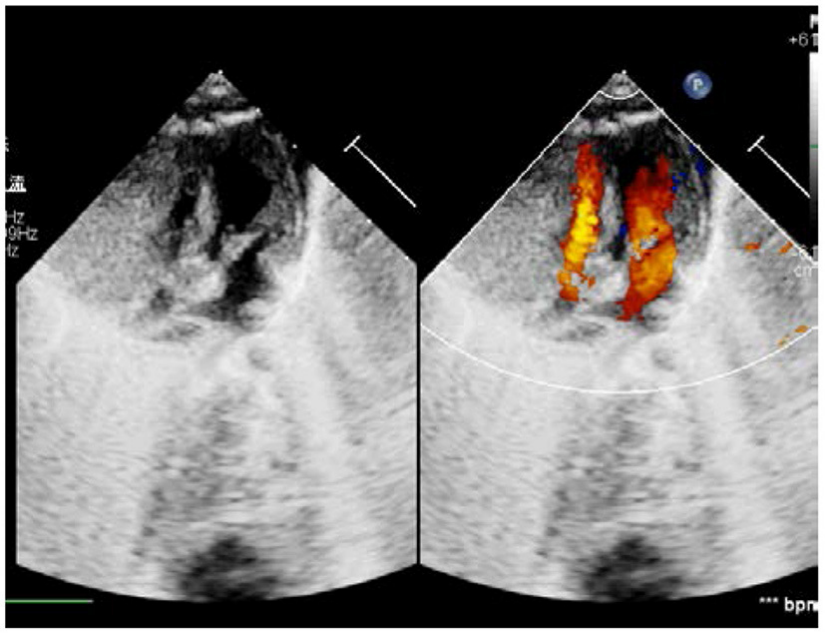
Ultrasonic Cardiogram: Neoplastic mass on the right ventricular wall and constricted right cardiac cavity.
